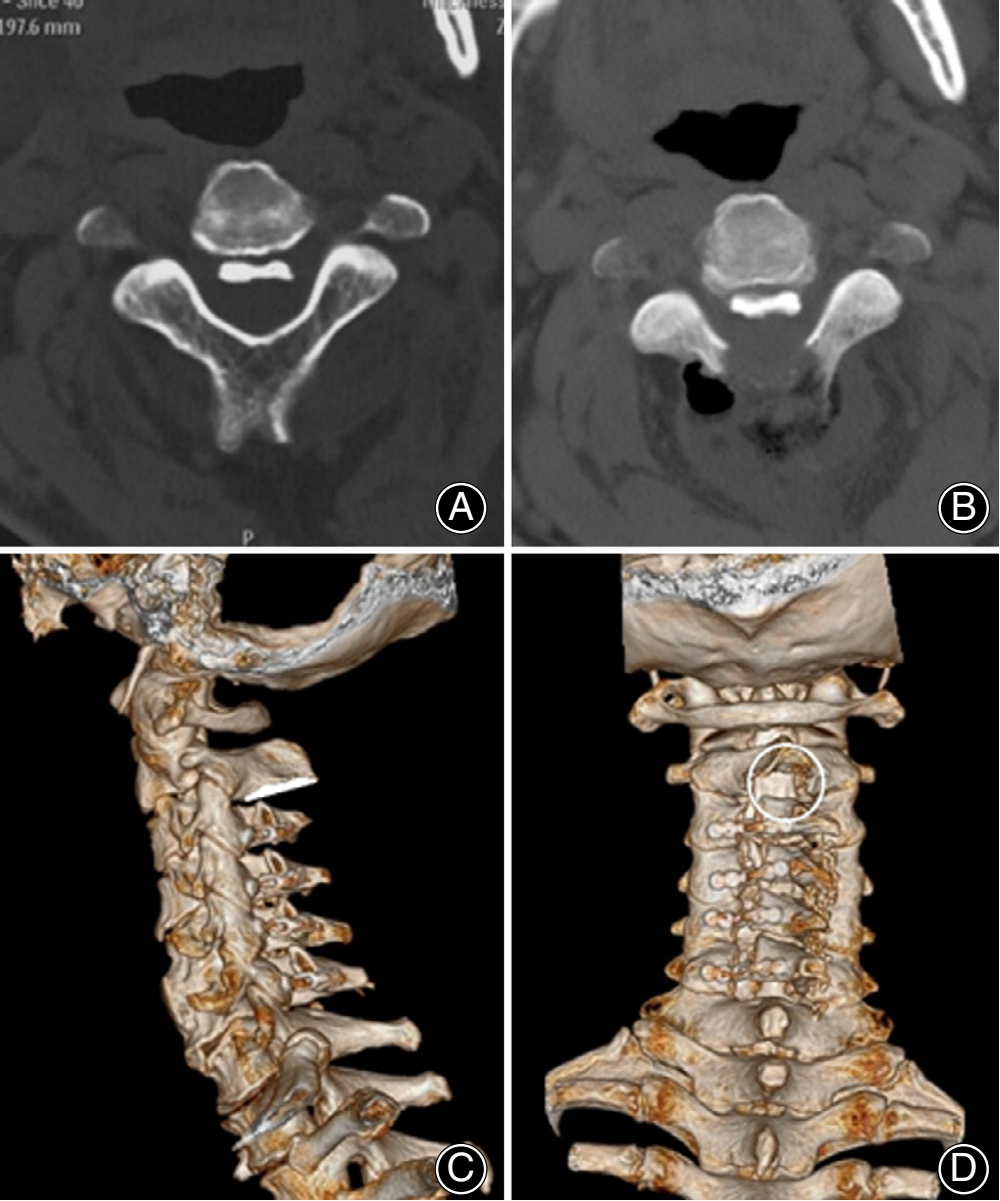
CT scans showing the area of dissected C_2_ lamina. The upper two figures showing the same section before (A) and after (B) of the ultrasonic curette‐assisted laminoplasty in C_2_ level. The lower figures showing the reconstructed 3D view of the patient after the curette‐assisted laminoplasty (C, D). Note that resection of flavum ligament is necessary to certify that there has been sufficient space for the spinal cord to shift after the procedure (area is indicated with a white circle in CT reconstructed image in D).
